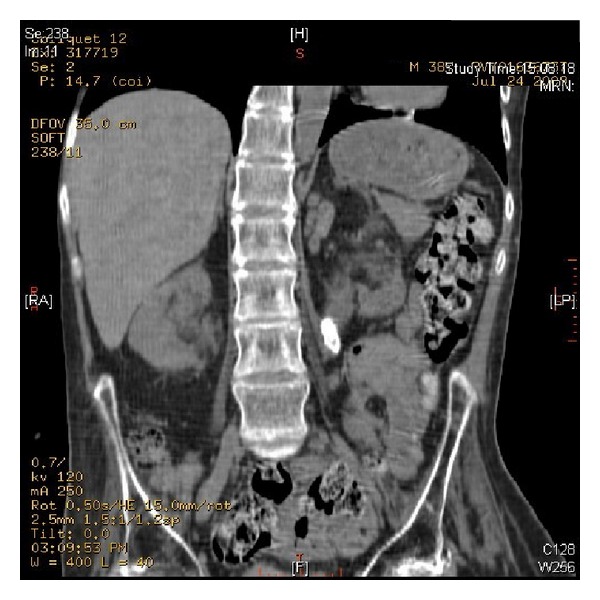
CT of urinary tract, performed on 24 July 2009, revealed two cm length calculus in left ureter at the level of L-3/4 causing mild hydronephrosis.
